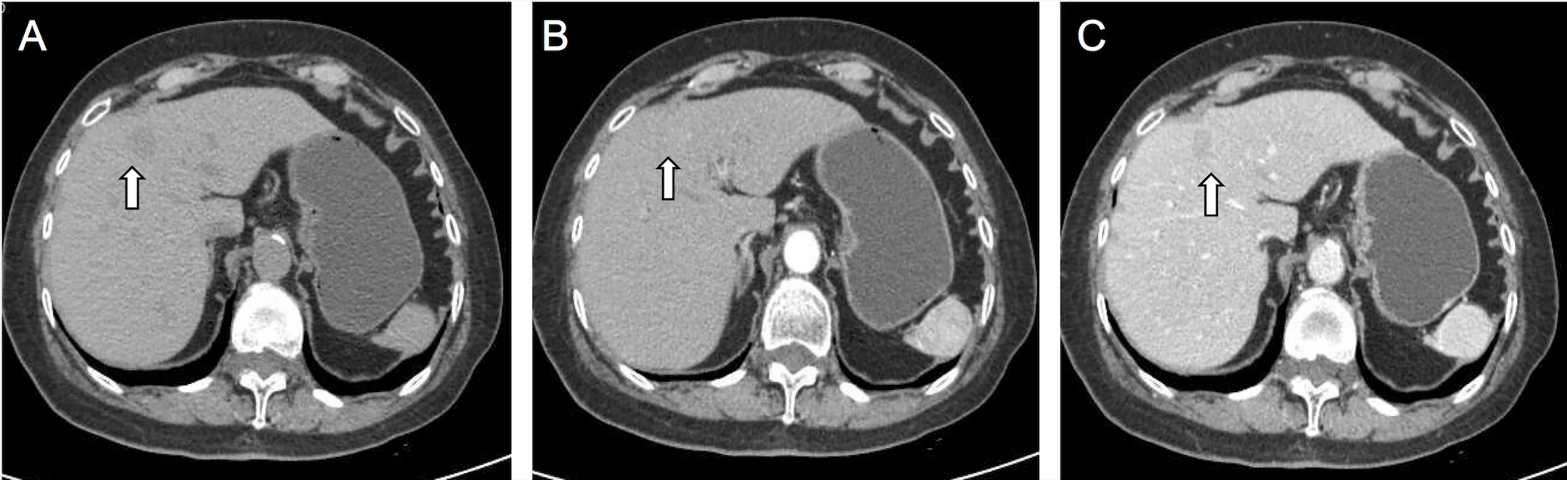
On CT plain image, the nodule is slightly low-density lesion in the S4 of the liver (A). On contrast-enhanced CT images, the lesion showed slight enhancement in arterial phase (B) and slight washout in portal phases (C). The white arrow points to the lesion. Malignant tumor could not be excluded.
